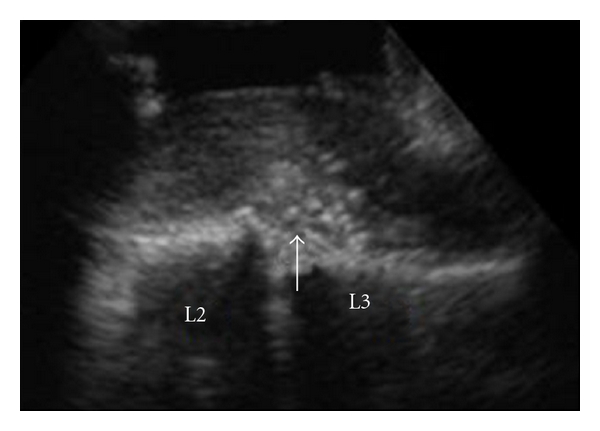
Intraoperative ultrasonography. Disc herniation was discovered in the dura (white arrow).
